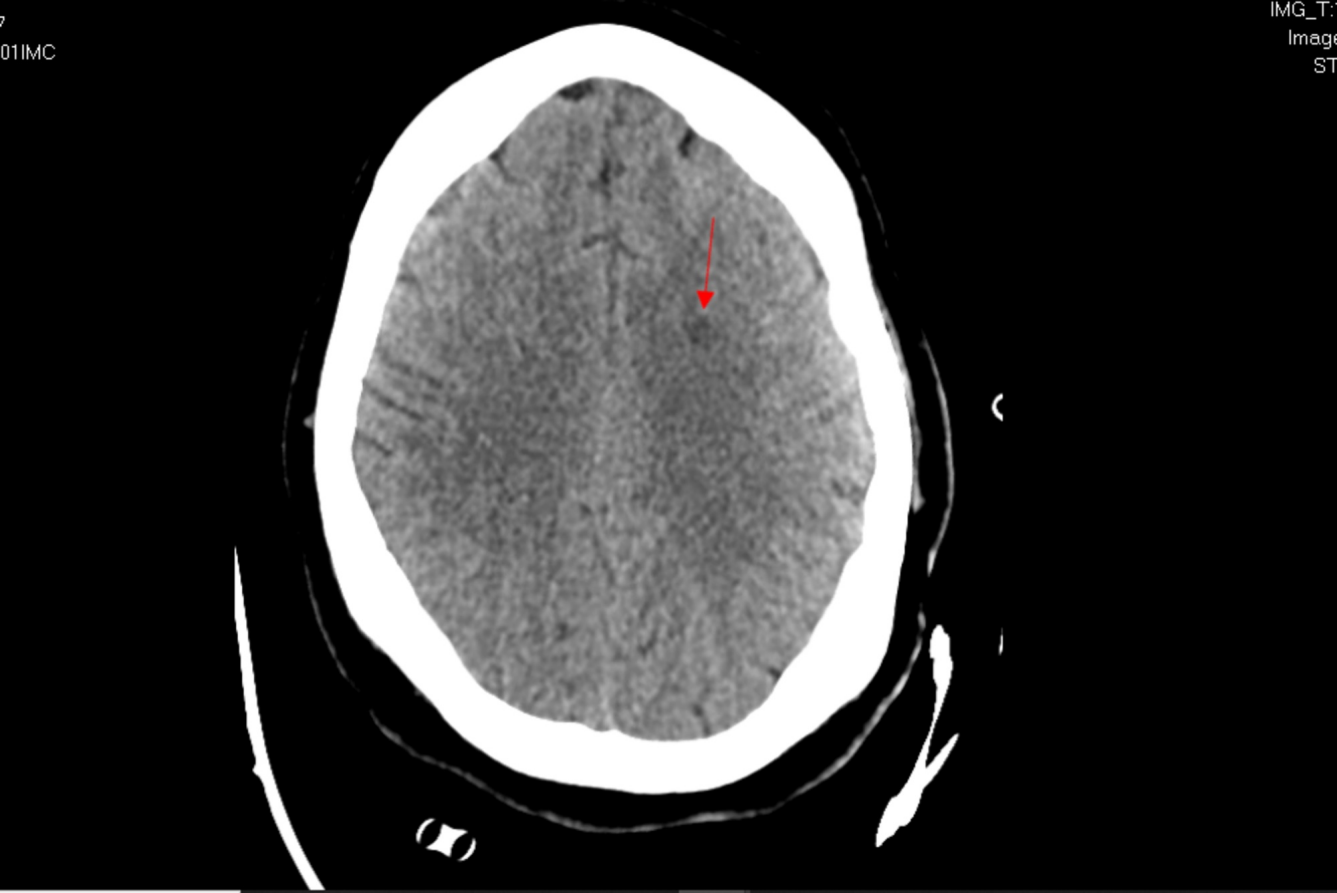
Case 1 repeat head CT on April 21, 2020
